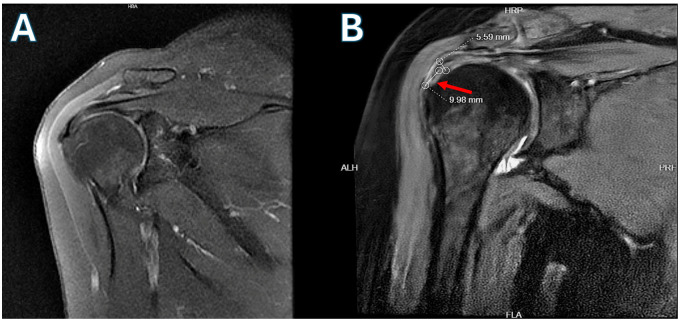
Representative MRI findings of the supraspinatus tendon (SST). (A) Normal SST demonstrating preserved tendon continuity and normal signal intensity. (B) Rotator cuff tear (RCT) showing disruption of tendon continuity with increased intratendinous signal intensity (arrows).
